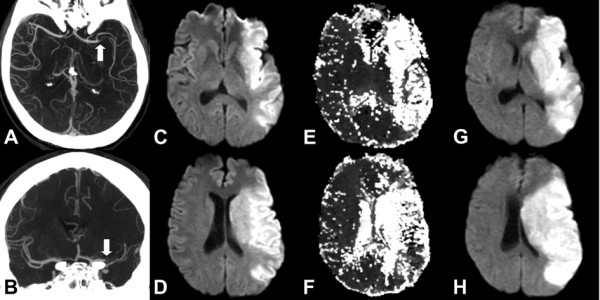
51 year old male presenting to the ED with right hemiparesis and aphasia. Imaging was initiated 4 hours after witnessed symptom onset. A &B. CTA demonstrates occlusion of the proximal left MCA (white arrows). C &D. Initial DWI shows large abnormality of the left MCA territory. E &F. MR perfusion time to peak (TTP) maps demonstrates hypoperfusion of similar extent as the diffusion abnormality. G &H. Follow-up MRI shows no significant enlargement of the initial abnormality. Whole brain map volume measurements for this patient are: DWI: 154 ml; MTT: 211 ml (mismatch: 57 ml); TTP: 241 ml (mismatch 87 ml).
